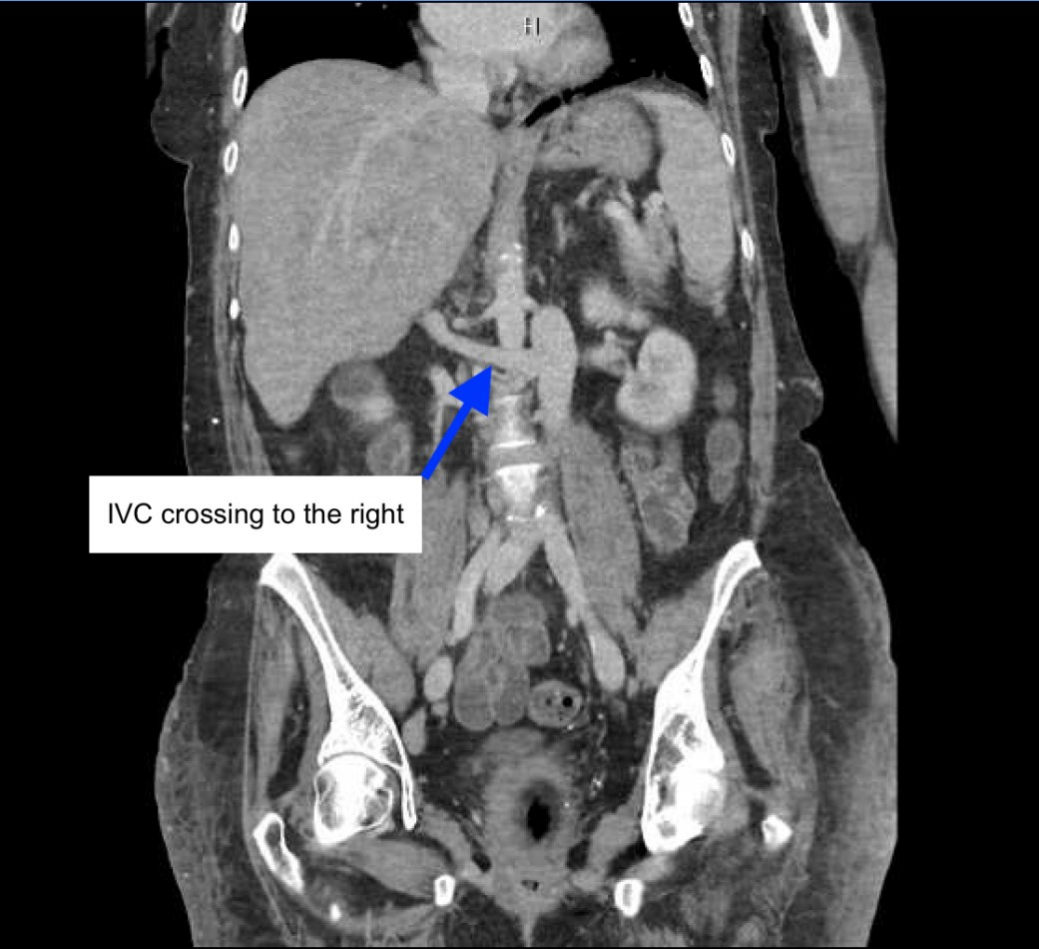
L sided IVC crossing to the right anterior to the aorta. (CT abdomen/pelvis w/contrast - coronal view).
